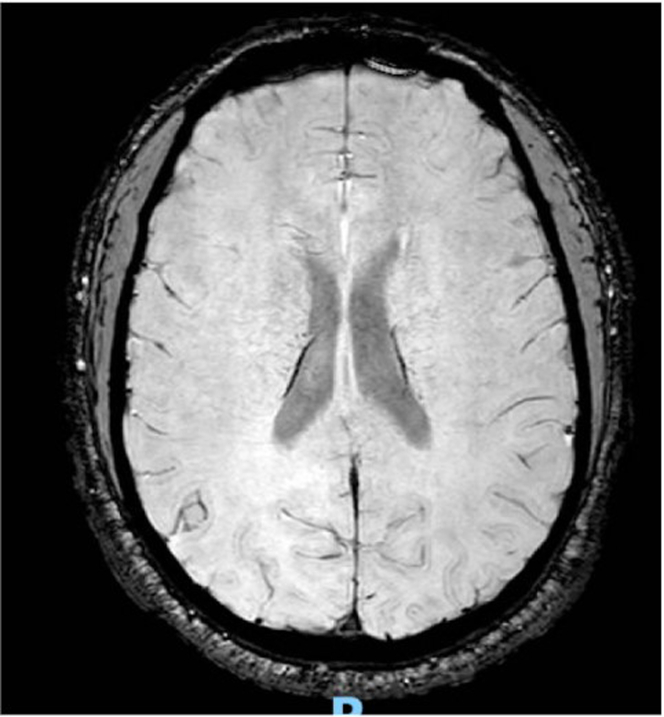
Brain MRI (T2-Weighted Axial Sequence) MRI of the brain demonstrating no evidence of acute infarction, hemorrhage, or posterior reversible encephalopathy syndrome (PRES). MRI = magnetic resonance imaging.
